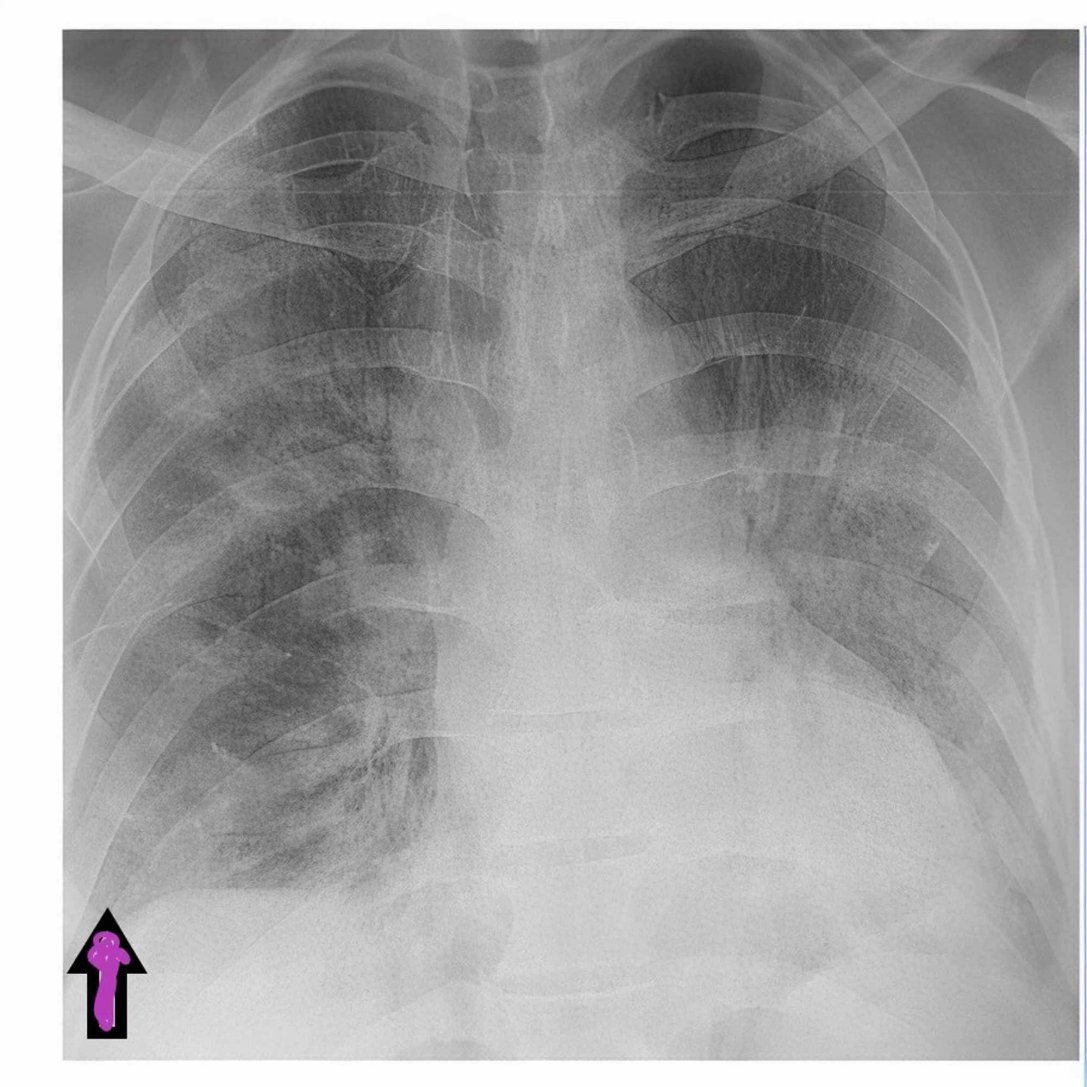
Chest X-ray on admission. Imaging reveals mild bilateral lung infiltrates. Purple arrow represents clear lung angle with no pneumothorax or pneumomediastinum.
